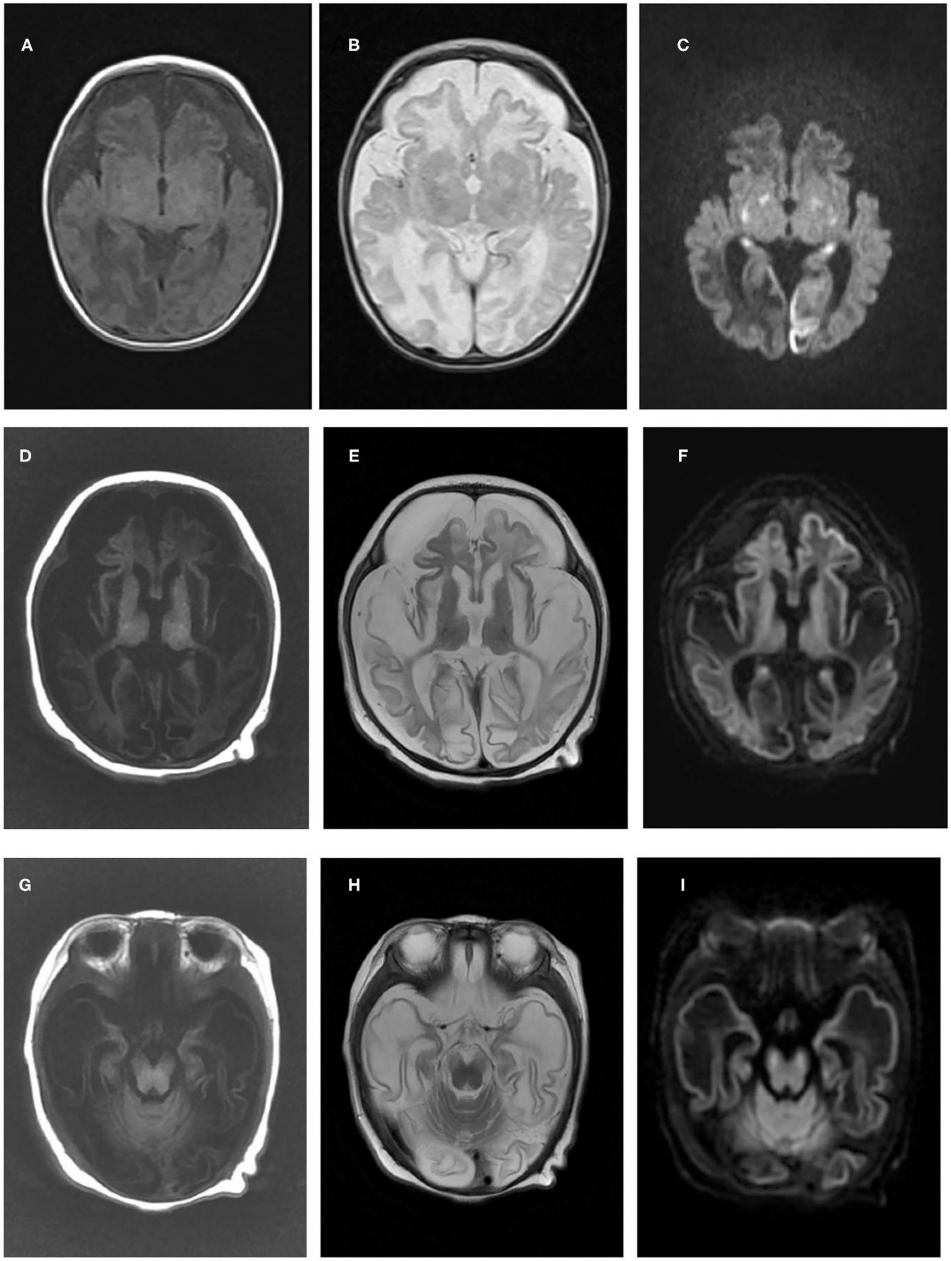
Brain magnetic resonance imaging results of the two patients in the present study. Brain magnetic resonance imaging of patient one in our study at 1 month old revealed cerebral atrophy and partial encephalomalacia, a diffuse abnormal signal in the cerebral white matter, with a low signal in T1WI (A) and a high signal in T2WI (B), and high signals in the bilateral occipital and temporal lobes, basal ganglia and thalamus (C). An MRI of patient two at 3 months old indicated polycystic encephalomalacia and diffuse cerebral atrophy, with diffuse abnormal signals in the brain, low signals on T1WI and high signals on T2WI and DWI (D–F), and a high signal in the cerebral peduncle (G–I).
